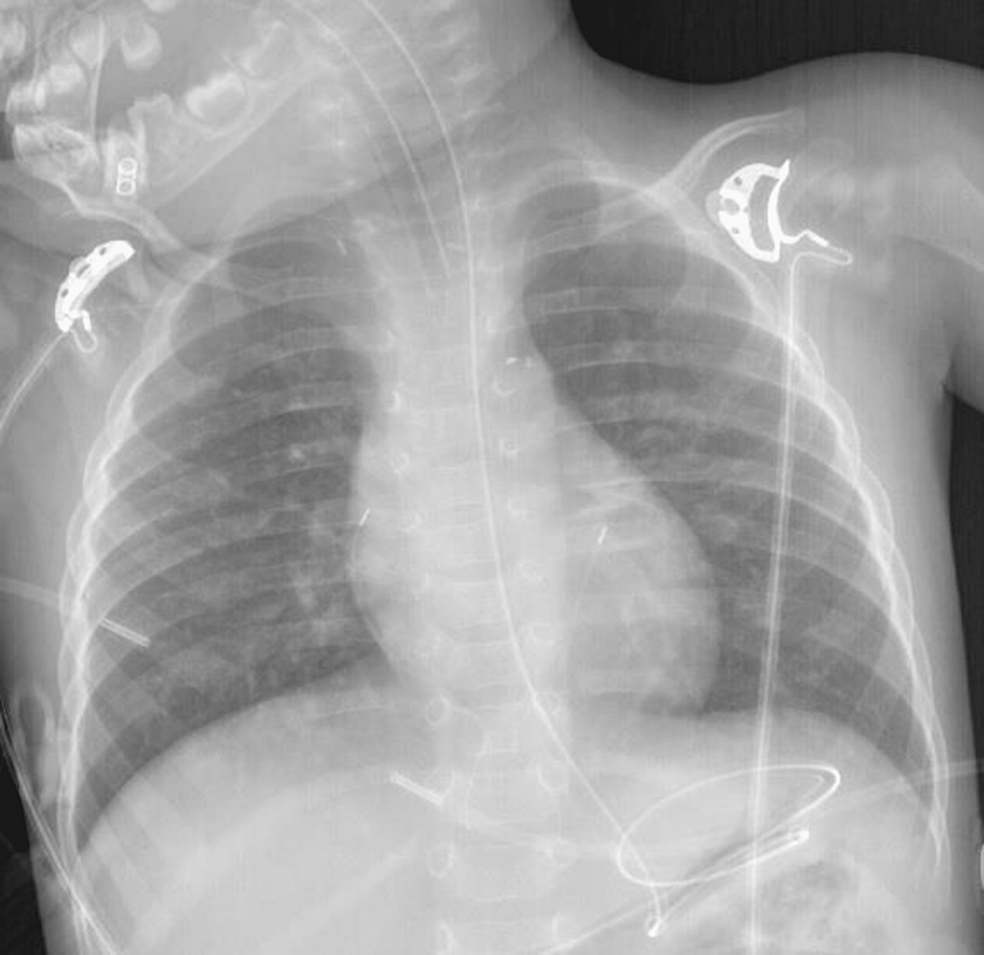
Radiographic evaluation of endotracheal tube position The endotracheal tube was correctly placed. In this chest radiograph, tracheal stenosis is not apparent.
